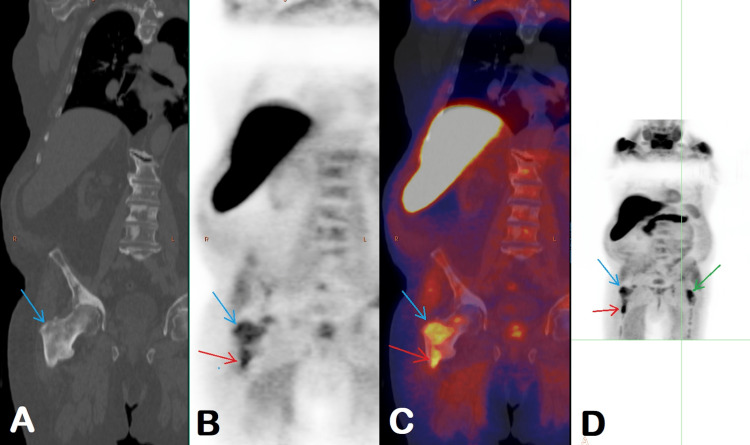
Findings on a fluoride-18 fluciclovine PET-CT scan in April 2022 CT (A), PET (B), fused PET/CT (C), and MIP (D) images show an increased number of foci of abnormally increased radiotracer activity in the skeleton (red arrows). There is an increased extent of sclerosis and associated abnormal radiotracer activity in the intertrochanteric region of the right femur (blue arrows). Activity in the previously seen lesion decreased to a SUVmax of 3.5 from a previous SUVmax of 4.1 (for reference, background bone marrow activity SUVmax of 2.8). Activity in the new area of sclerosis is SUVmax of 5.5. The new focus of abnormal radiotracer uptake was seen corresponding to the faint sclerotic lesion in the left femoral neck (green arrow) with a SUVmax of 5.5. PET – positron emission tomography, MIP – maximum intensity projection, SUVmax – maximum standardized uptake value
